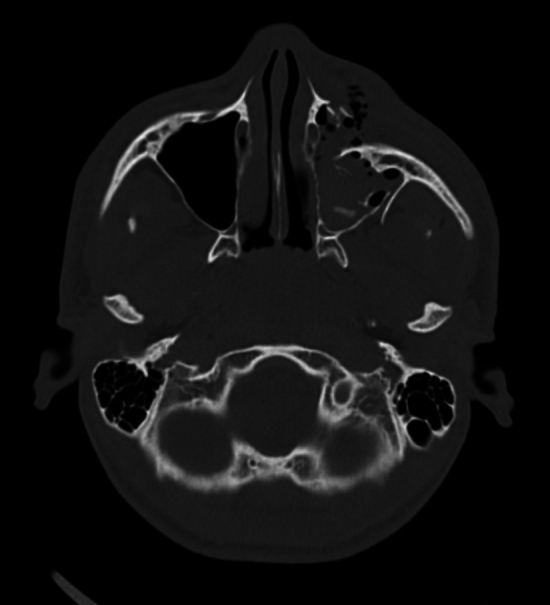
Axial bone window CT image of a 16-year-old player with a displaced left zygomatico-maxillary complex fracture
